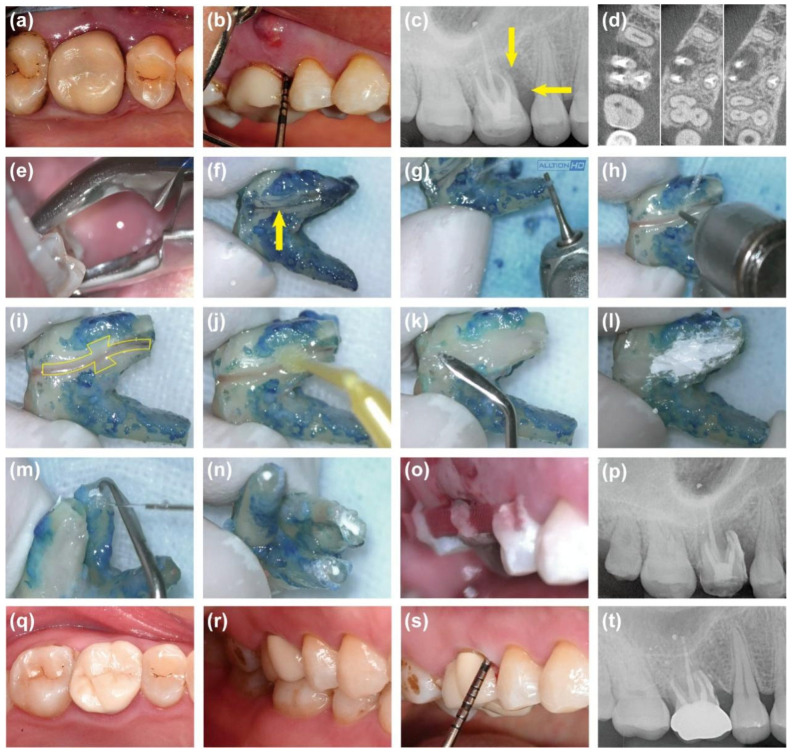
Photographs and radiographic images of case 2. (a,b) Pre-operative intraoral photographs showing sinus tract and deep periodontal probing depth. (c) Pre-operative radiograph showing the radiolucent area (arrows). (d) Pre-operative CBCT. (e) Extraction of Tooth #16. (f) Methylene blue dying showing fracture line (arrow). (g) Excision of the 3 mm root apex. (h) Fracture expanding. (i) Preparation of trapezoidal retention forms on both sides of the fracture. (j) Application of bonding agent. (k) Filling of the fracture and retention forms with resin. (l) Coverage of the resin surface with iRoot BP Plus. (m) Retrograde preparation. (n) Retrograde filling. (o) Replantation. (p) Immediate post-operative radiograph. (q–s) Intraoral photographs at 21 months showing normal periodontal appearance and probing depth. (t) Periapical radiograph at 21 months showing periradicular healing.
